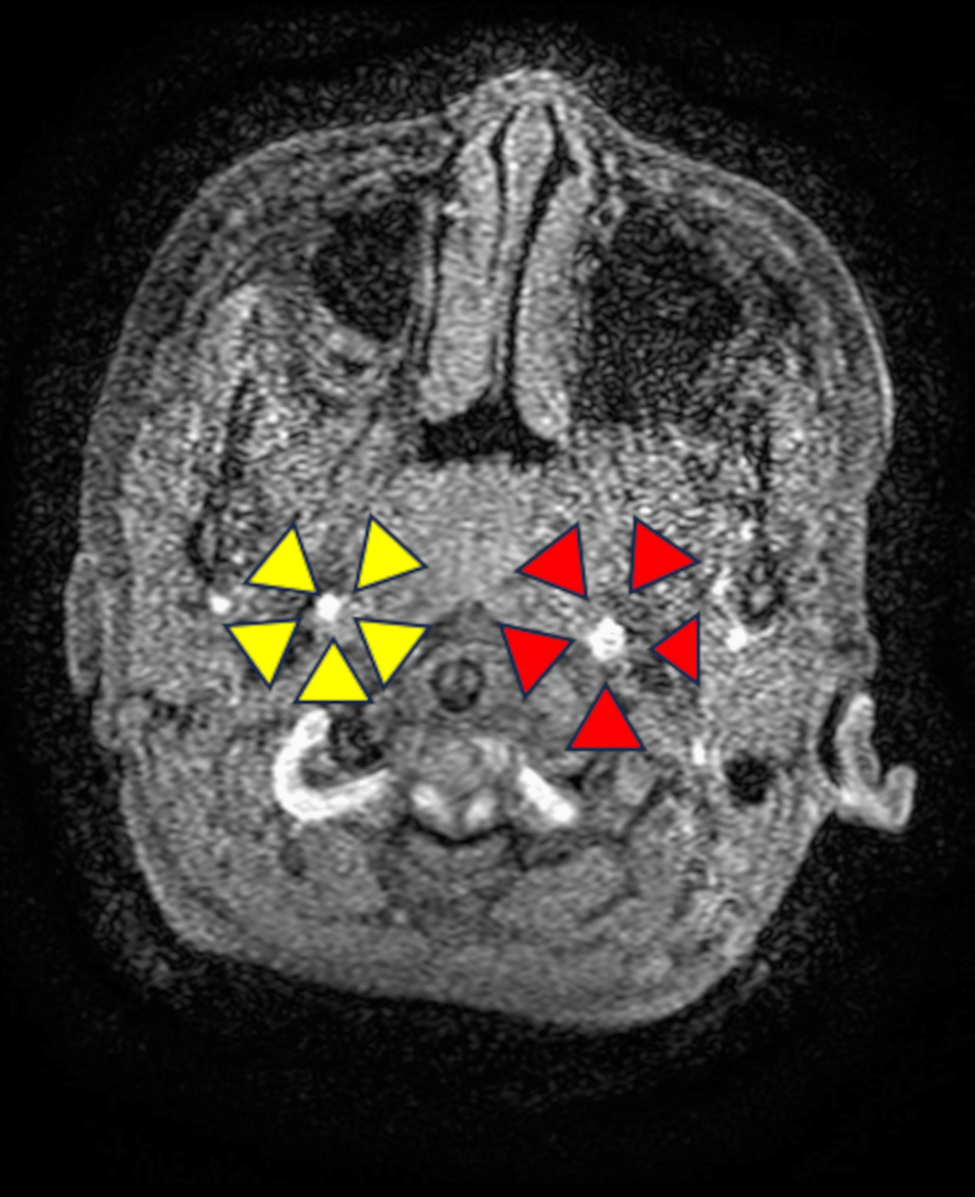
Magnetic resonance angiography of the head on day 12 of admission. On day 12 of the patient's hospitalization, a head MRI angiogram showed a difference in the diameter of the middle cerebral artery on the left and right sides. The diameter on the right (yellow arrowheads) was smaller than that on the left (red arrowheads), indicating a narrowing of the lumen of the artery.
